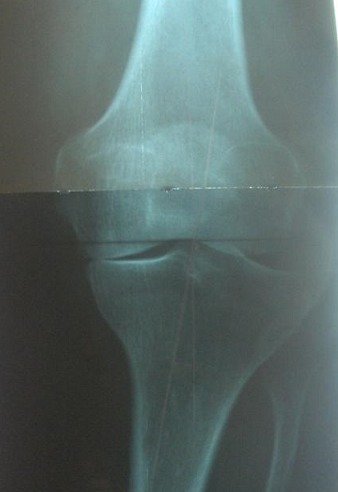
Radiographie du genou gauche en charge chez un patient présentant un genu varum de 15° avec pincement de l'interligne avec une arthrose du compartiment interne stade II d'Ahlback
